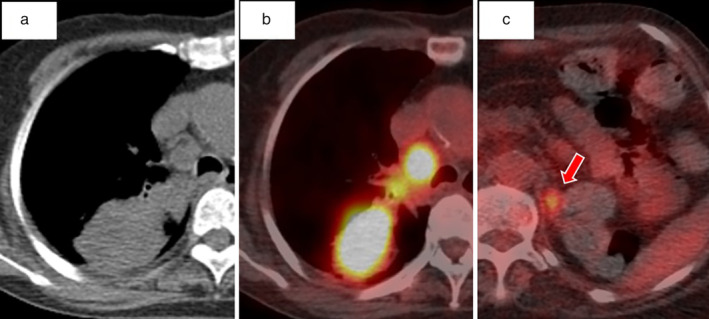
(a) Chest unenhanced computed tomography (CT) scan at hospitalization revealed a tumor shadow in the upper lobe of the right lung. Positron emission tomography (PET)‐CT scan before chemotherapy showed SUVmax: (b) 26.0 to the mass in the upper lobe of the right lung, and (c) 4.8 in the left adrenal gland of with ^18^F‐fluorodeoxyglucose (FDG) integration.
